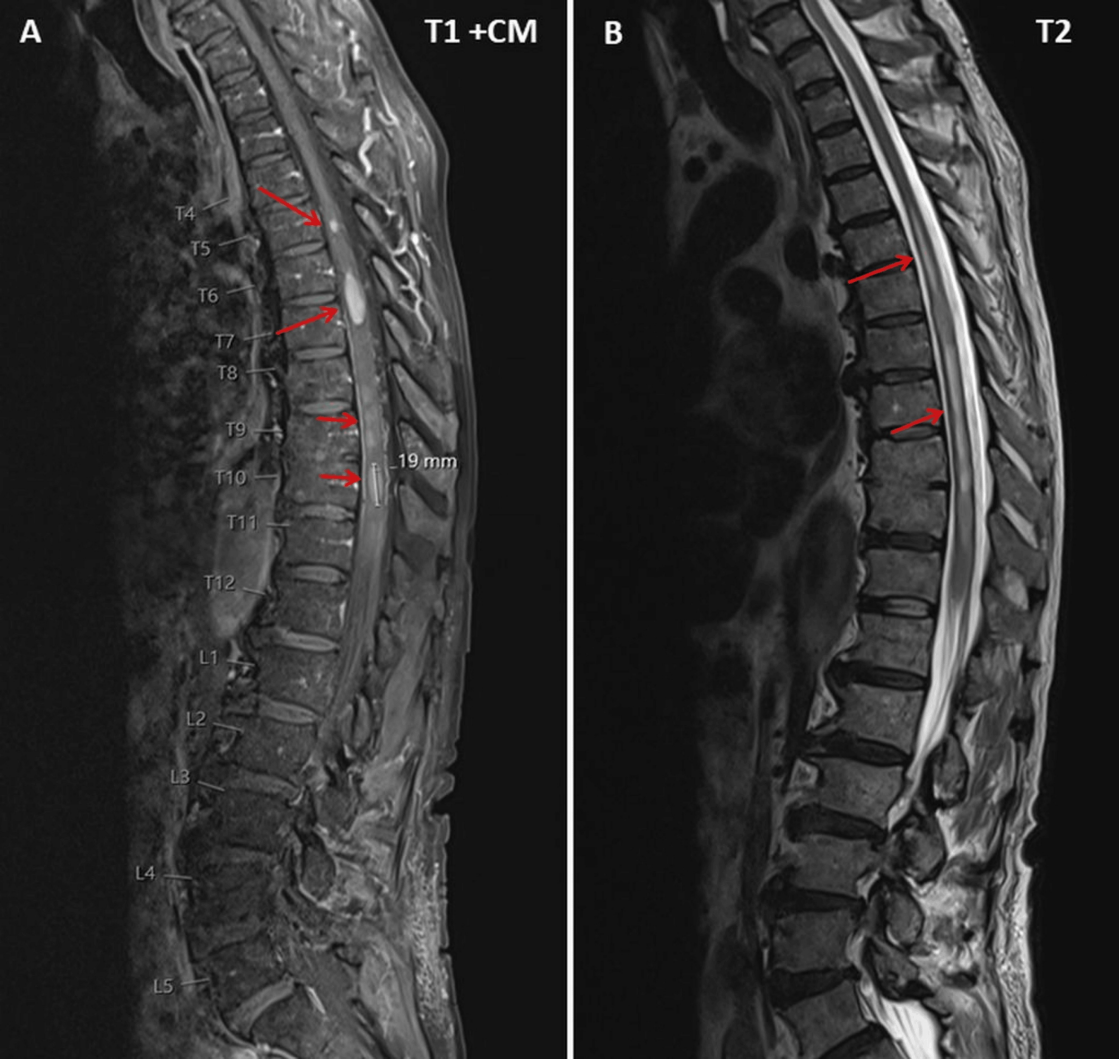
MRI of the thoracic and lumbar spine showing T1 and T2 hyperintense lesions with discrete contrast enhancement in the vertebral bodies of T6, T10, and T11 and multiple oval- to pinhead-shaped, eccentric lesions in the spinal cord at T5 and T11 (panel A, arrows), with accompanying hydromyelia between T4 and T10 (panel B, arrows) MRI: magnetic resonance imaging
